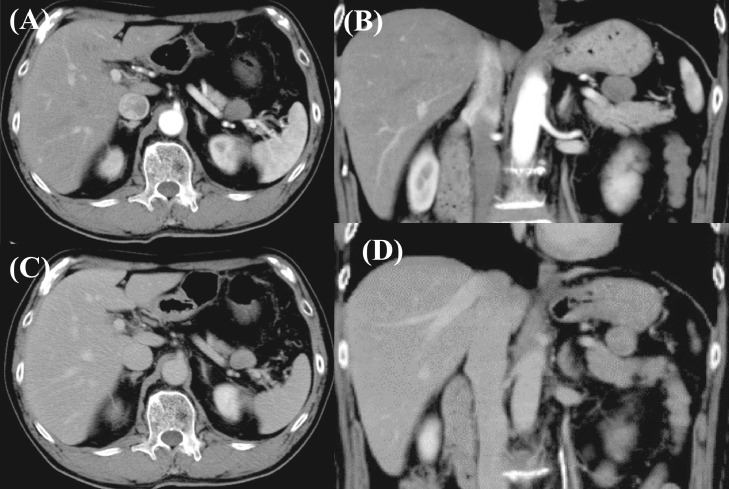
Contrast-enhanced CT findings 4 months after initial CT. The tumor had grown to 19 × 23 × 20 mm in diameter. In early phase, the mass was poorly enhanced on axial sectioning (A) and coronal sectioning (B). In late phase, the mass was enhanced and similar to pancreas on axial sectioning (C) and on coronal sectioning (D).
